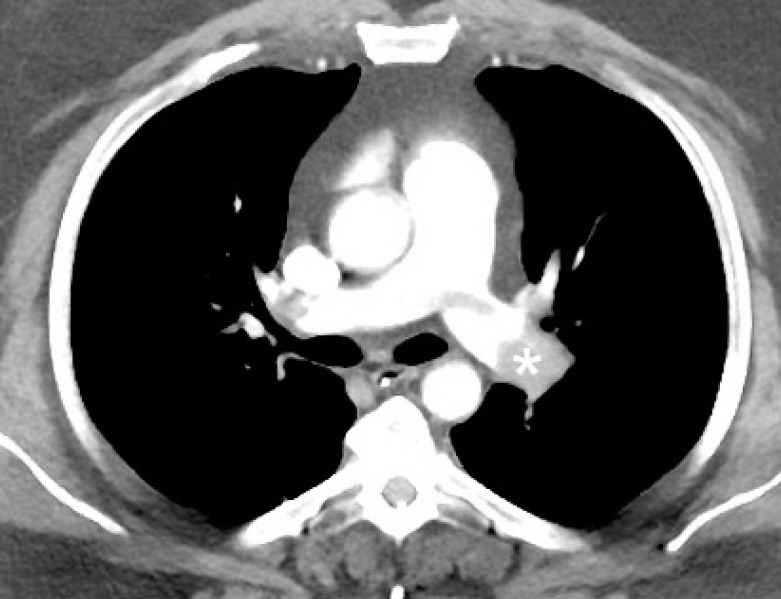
Post contrast image of CT chest of an elderly male on day 2 after a total knee replacement shows a filling defect in the left pulmonary artery (asterisk) suggesting pulmonary embolism. A small filling defect is also noted in the right pulmonary artery
